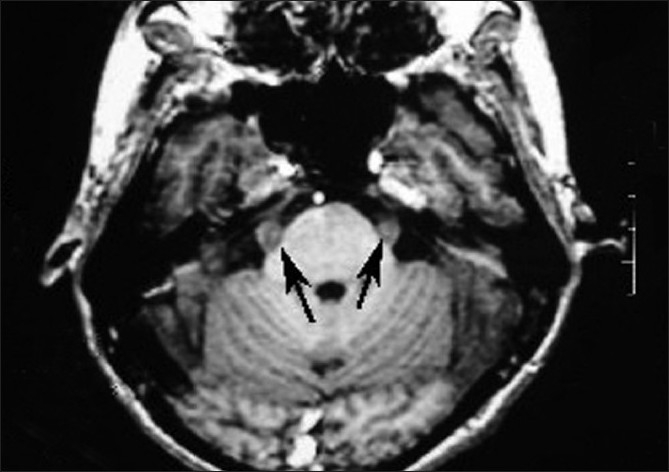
T1-weighted image showing thickened trigeminal nerves bilaterally as shown by arrows
